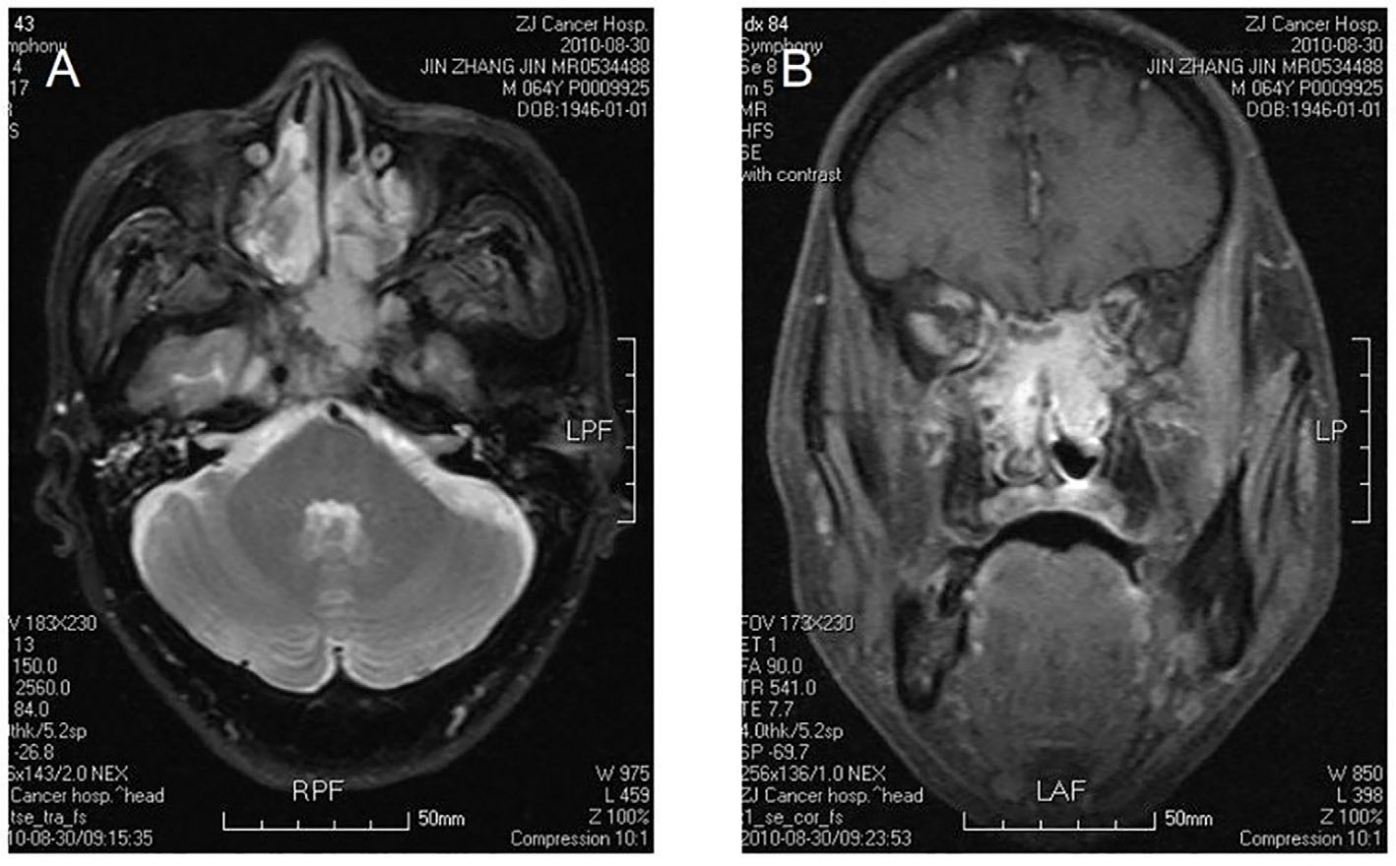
Bilateral nasal cavity and sinus osteosarcoma. MR image shows bilateral postirradiation osteosarcoma in a mixture of nasal and paranasal sinuses. In magnetic resonance imaging, the signal was isointensity in T1 and high in T2 weighted images in bilateral postnaris, ethmoidal cellules and sphenoid sinus. The lesion violates medial orbital wall and musculus rectus medialis of left eye with irregular morpha. There was evident intensification after contrast. The signal of skull bone is abnormal (A, B).
